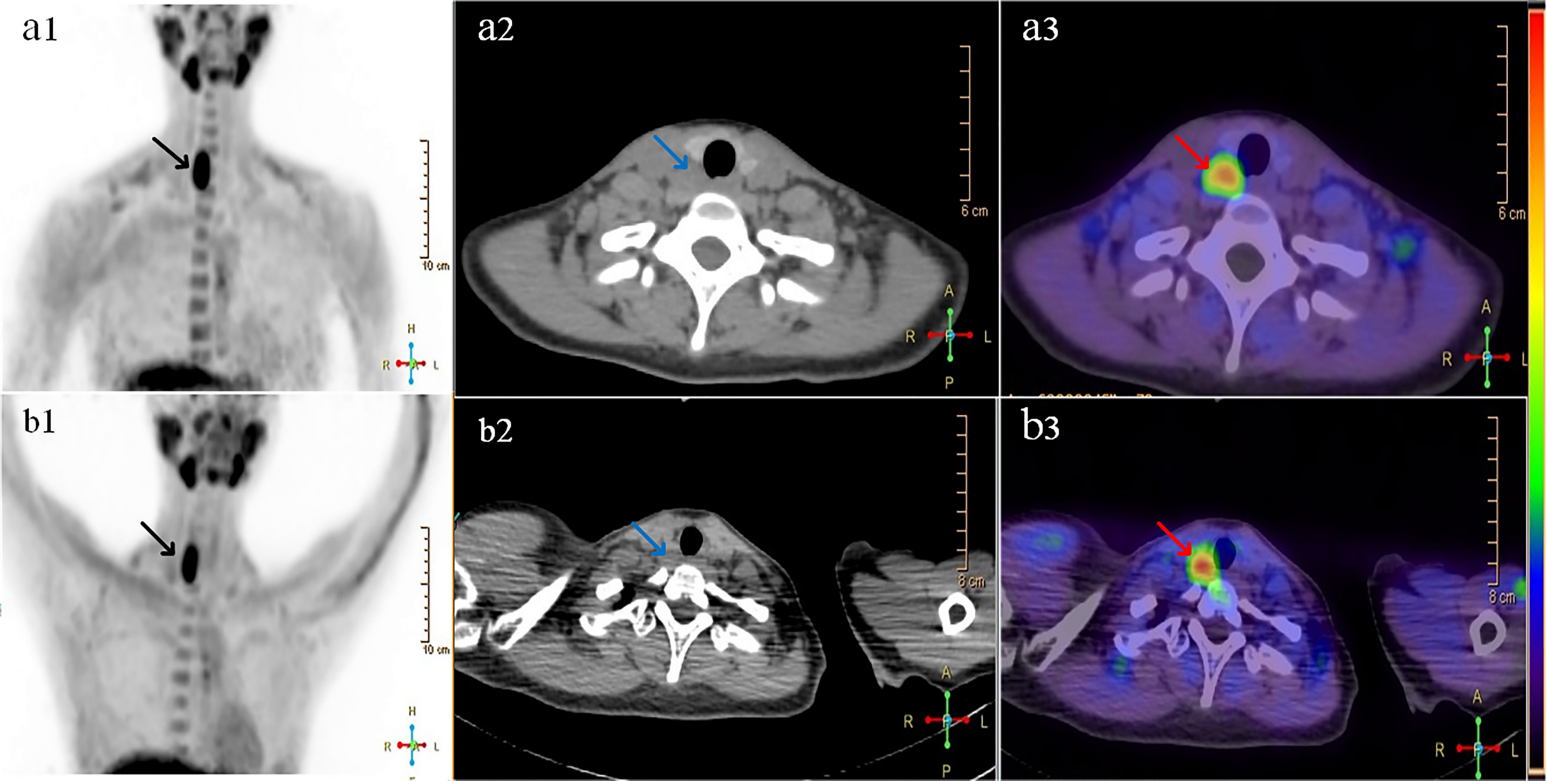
A 28-year-old woman with pain in the lower right back and fatigue of limbs for more than 3 months. The relevant inspection is as follows: PTH 577.3Pg/ml (12-88 Pg/ml), calcium 2.77mmol/L(2.15~2.5mmol/L), 25-hydroxyvitaminD 13.7ng/ml (>20ng/ml). Images named “(A) ”were 5min ^18^F-FCH imaging: A1 is 5min PET MIP, black arrow showed ^18^F-FCH radioactive uptake; A2 was neck CT, blue arrow showed the increased soft tissue density behind the right thyroid; A3 was PET/CT fusion image, red arrow showed the nodules of ^18^F-FCH metabolic activity, SUVmax was 7.09, SUVmax of adjacent thyroid tissue was 2.20, and P / T was 3.22. Images named “(B)” showed 60min 18F-FCH imaging: SUVmax of nodules in B3 was 8.81, SUVmax of thyroid was 1.90, and P/T was 4.64. Surgical pathology: <lower right parathyroid gland> parathyroid adenoma.
